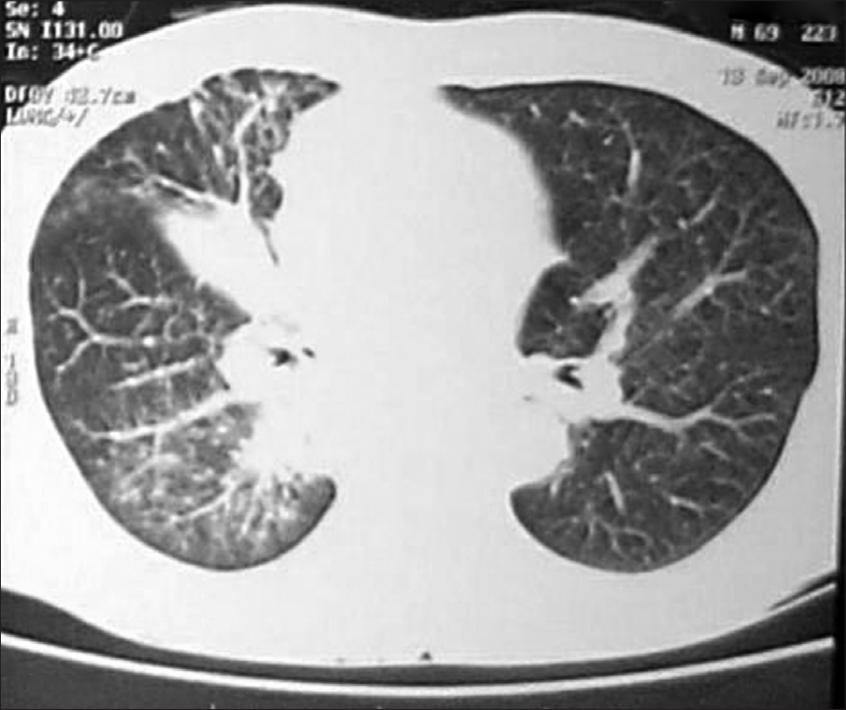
CT chest reveals ill defined soft tissue in the right hilar region encasing bronchus intermedius, causing right middle lobe collapse
